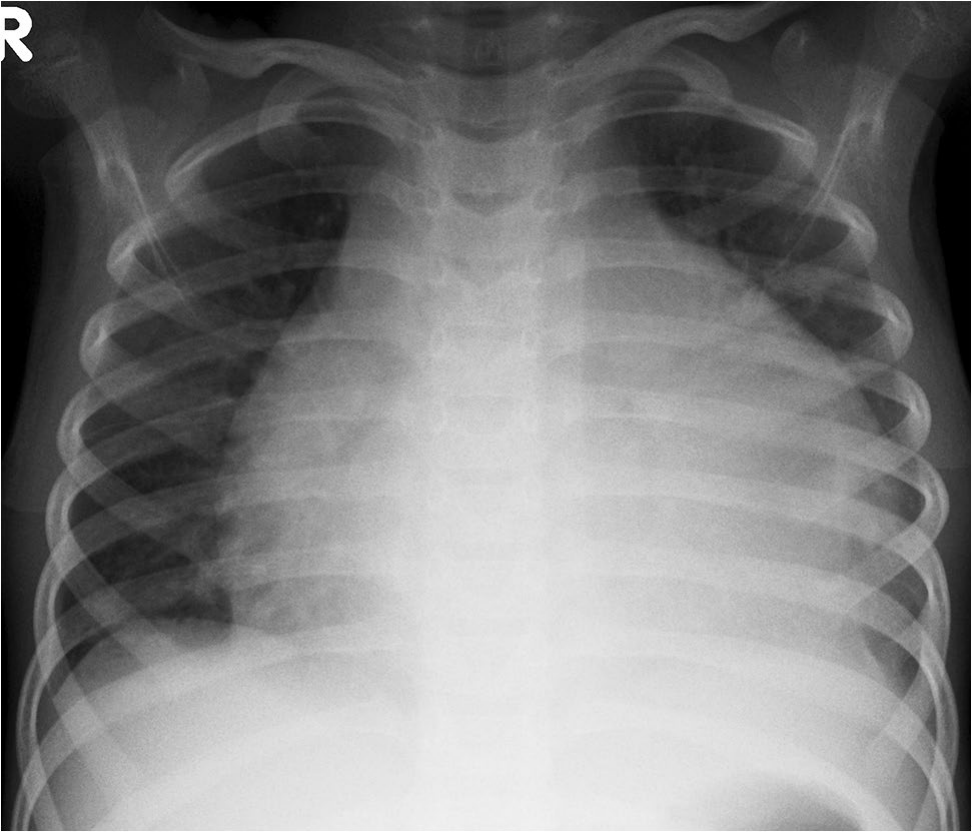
Posteroanterior chest radiograph in a 6-year-old boy demonstrates a tuberculous pericardial effusion  causing globular cardiomegaly
